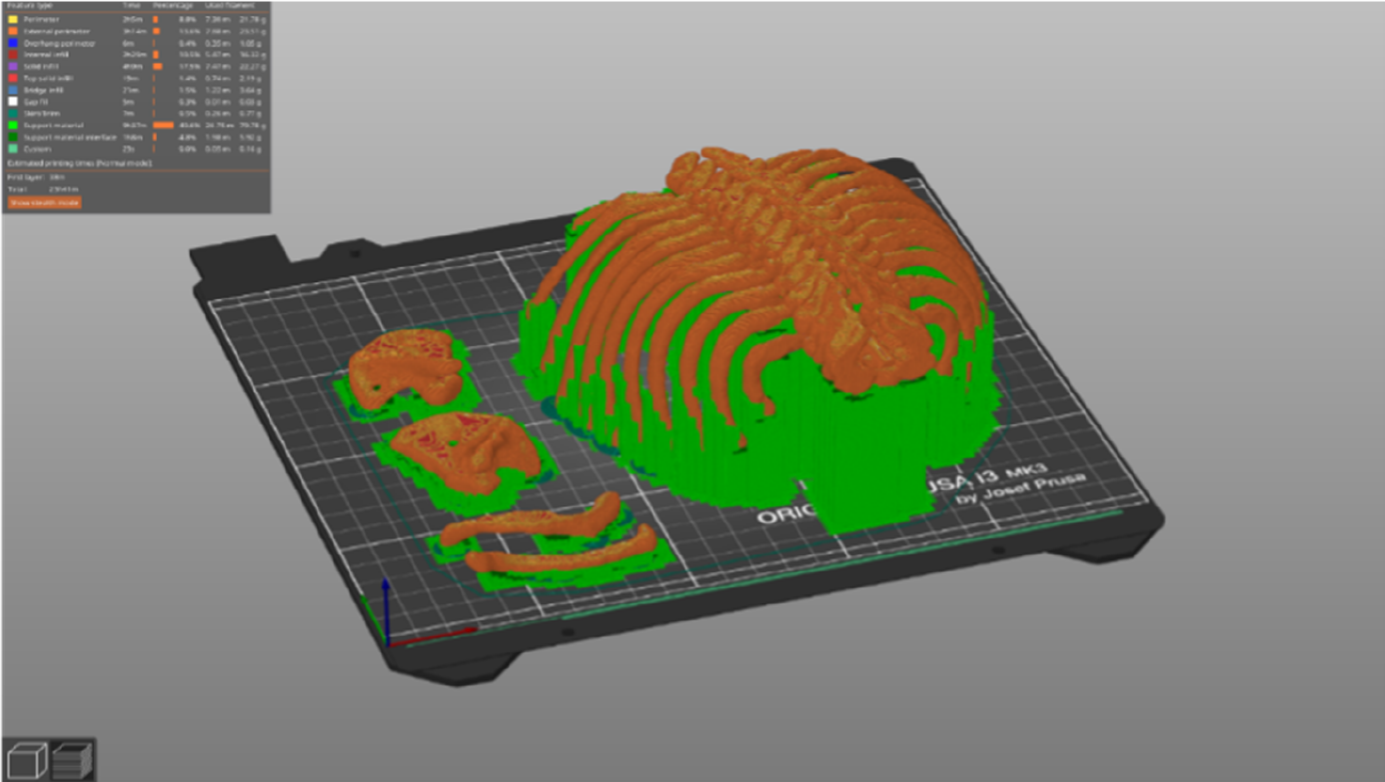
Process of segmentation: CT scan was utilized to segment a child's spinal column along with the ribcage, scapula, and clavicles using 3D slicer.
